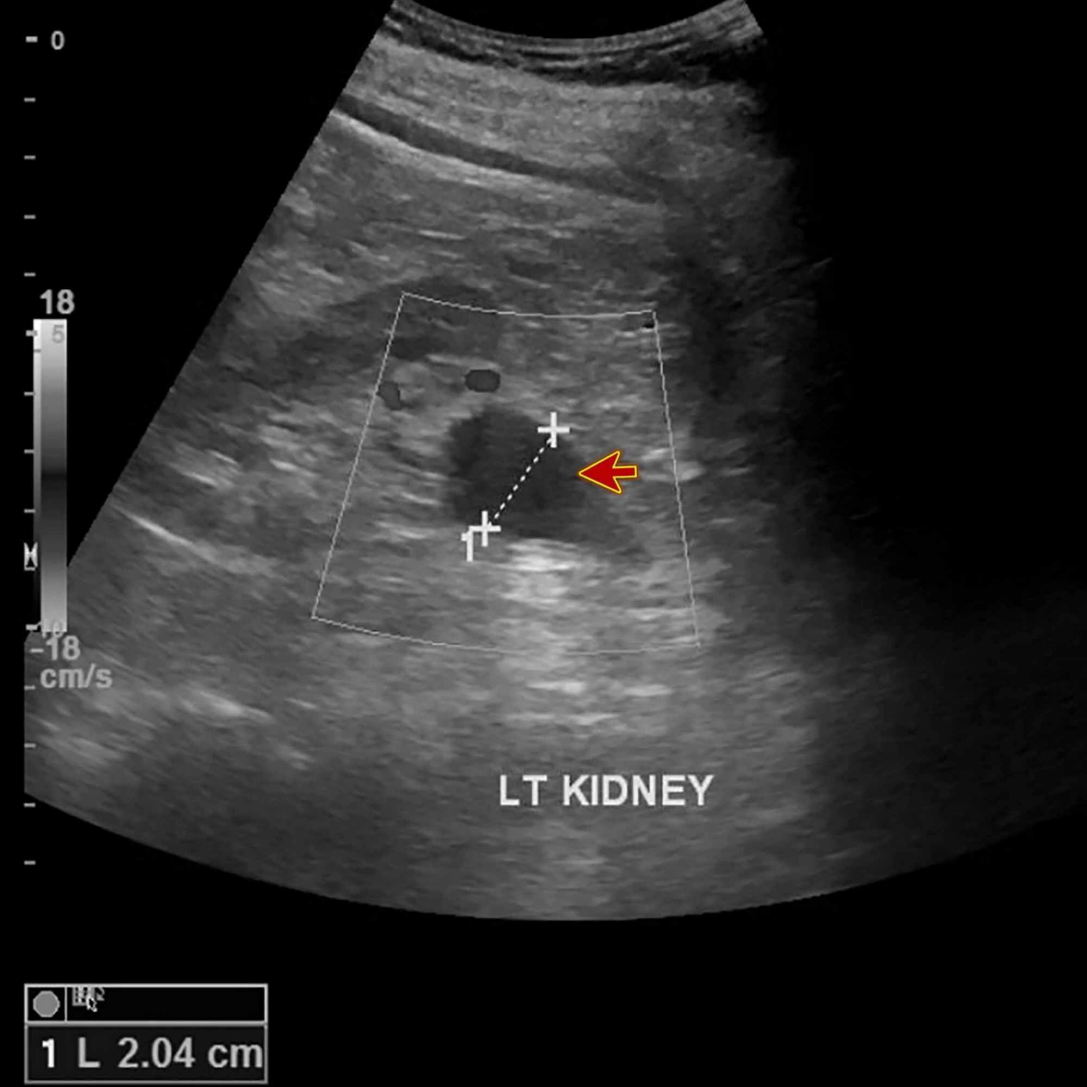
Bedside point-of-care ultrasound The image shows left kidney moderate hydronephrosis (red arrow)
